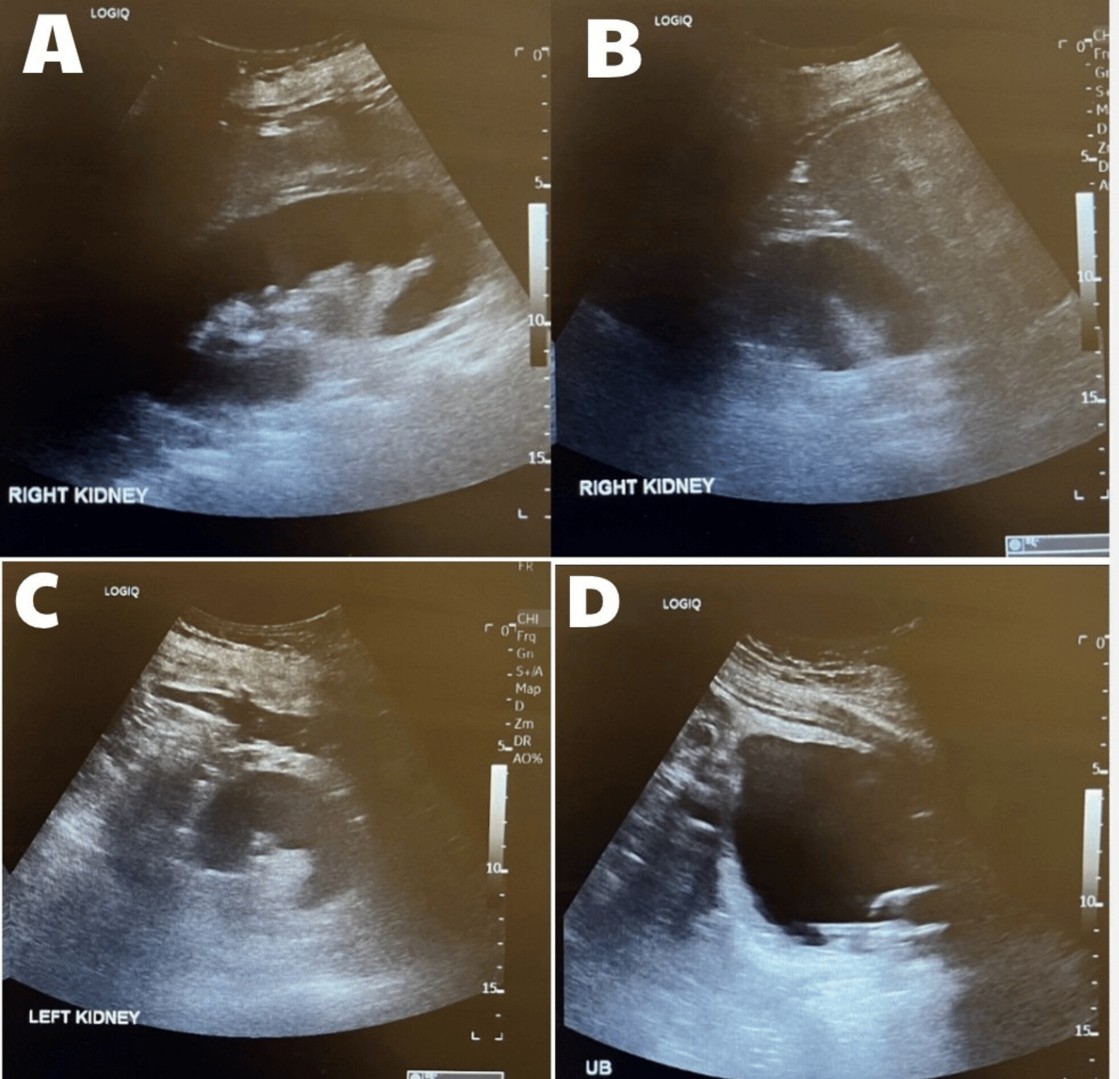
Ultrasound of the kidneys, ureters, and bladder (KUB) showing normal renal morphology with no evidence of hydronephrosis or obstructive uropathy Ultrasound of KUB demonstrating the right kidney (A, B), left kidney (C), and urinary bladder with catheter in situ (D).
